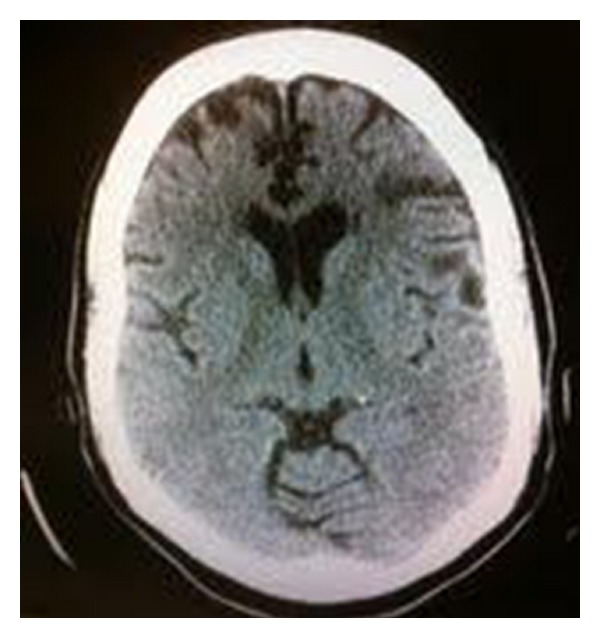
CT scan of the head without contrast showing old bifrontal encephalomalacia (left greater than right).
